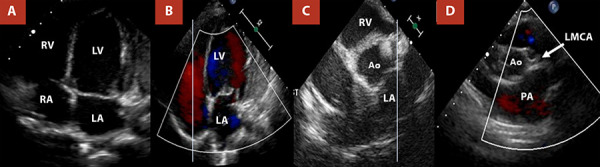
Follow-up transthoracic echocardiography at 2 years. (A) Reduction in left atrial size and decreased left ventricular diameter. (B) Minimal residual mitral regurgitation. (C, D) The left coronary trunk has been reimplanted into the left coronary sinus, with no diastolic reverse flow into the pulmonary artery.
